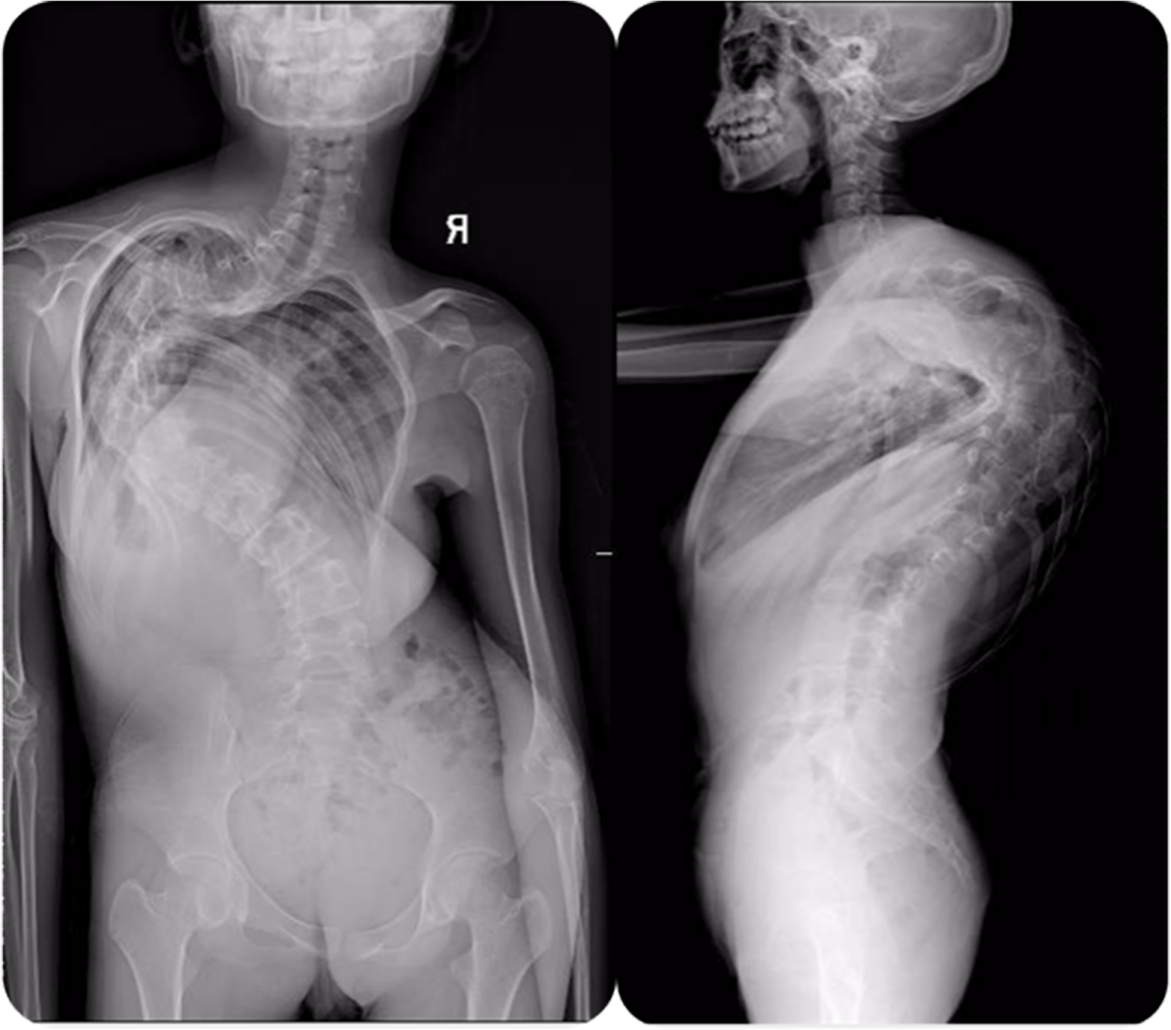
Preoperative standing, PA and lateral radiographs
